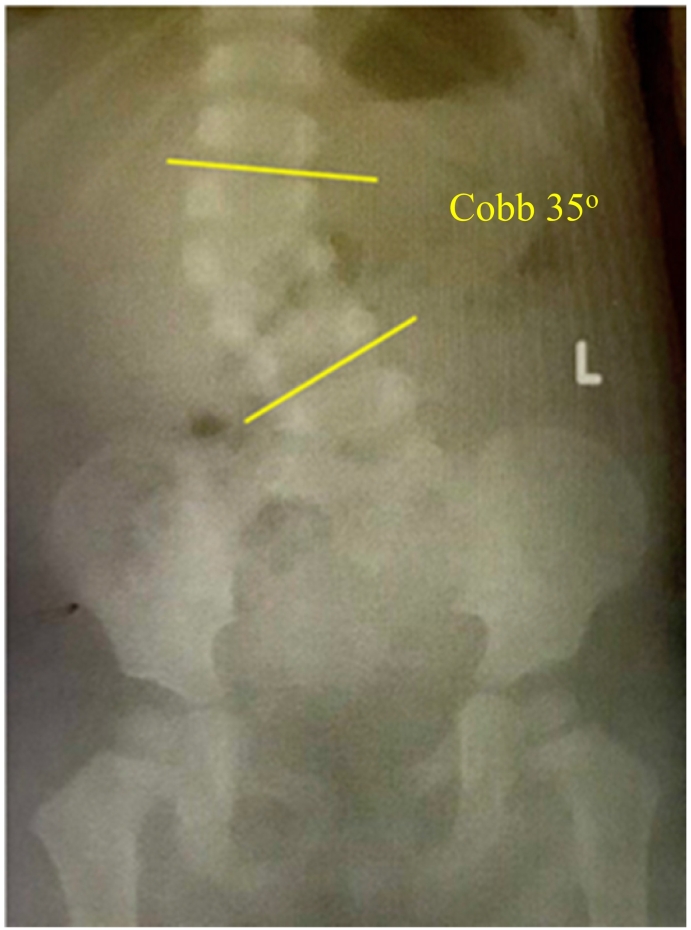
The X-ray of the patient before MSCs implantation showed hemivertebra on lumbar portion. The Cobb angle was 35 degrees.
